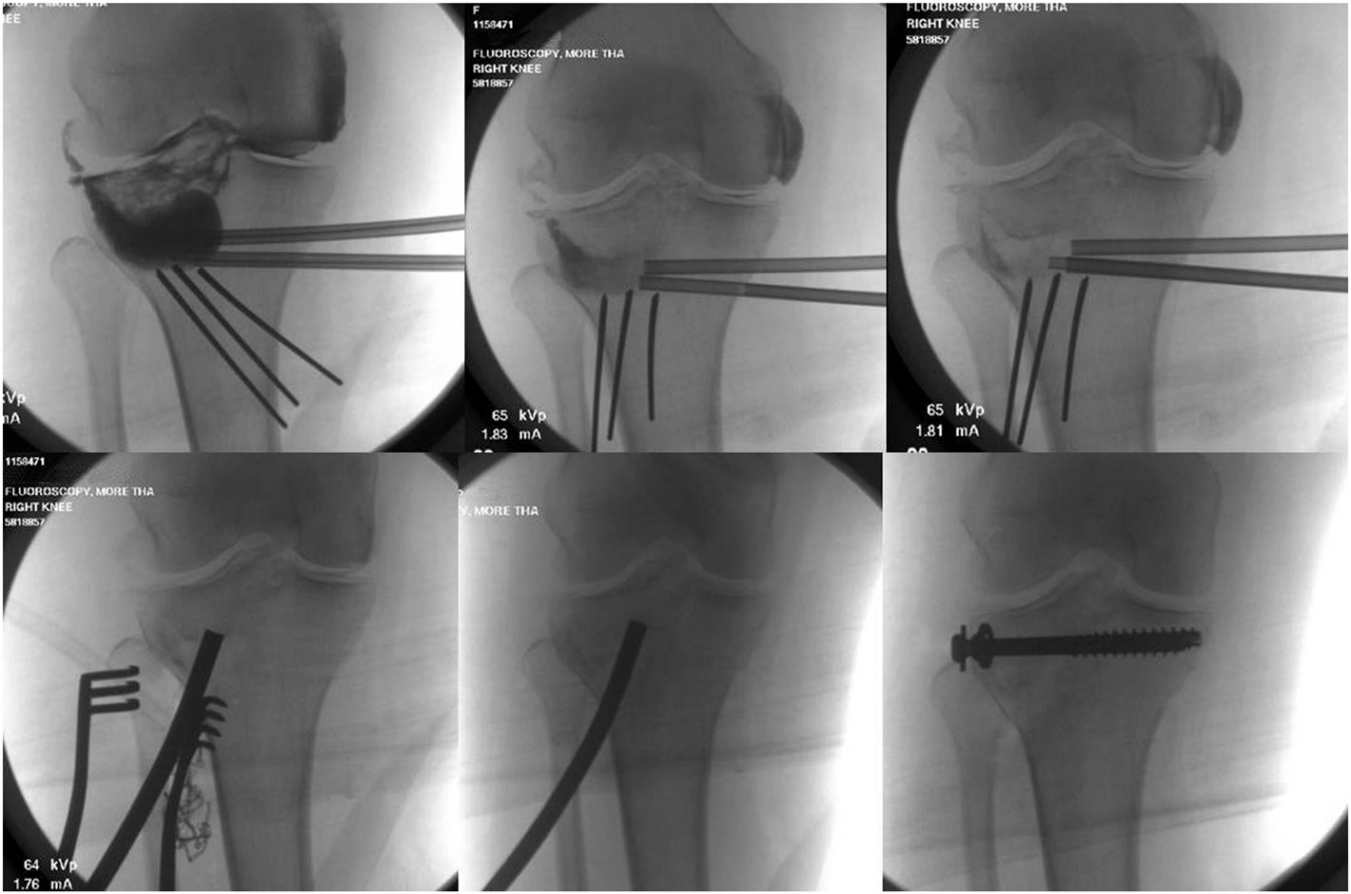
Intraoperative burst balloon with leakage of the radiographic contrast dye into the knee joint. This complication is easily resolved by a wash-out with 100-200 cc of sterile saline through the Jamshidi trocar. The bottom panels demonstrate the “bail-out” strategy for the irreducible articular fragment by conversion to the use of a conventional bone tamp and structured bone grafting.
